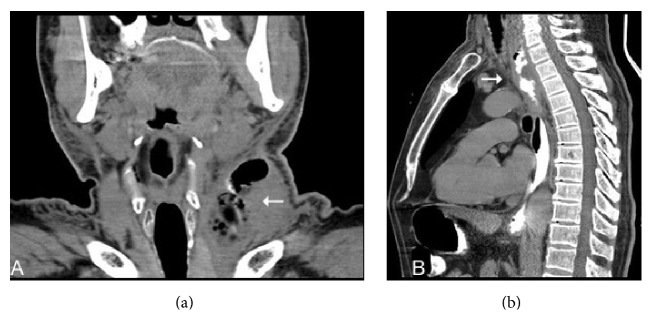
Computed tomography (CT) findings. (a) Inflammation and an abscess on the left side of the neck (arrow). (b) Inflammation of and contrast leakage into the left anterior mediastinum (arrow).
